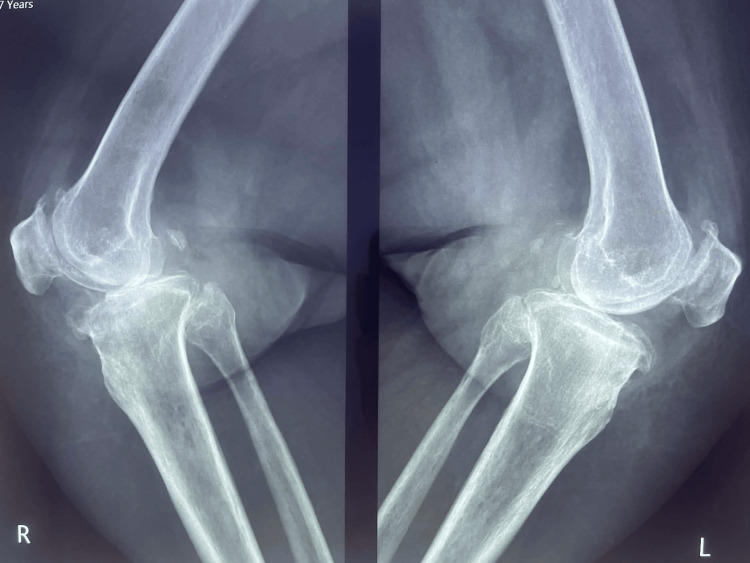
Preoperative lateral radiographs of both knees Preoperative lateral radiographs of both knees showing severe degenerative joint changes consistent with end-stage osteoarthritis.
